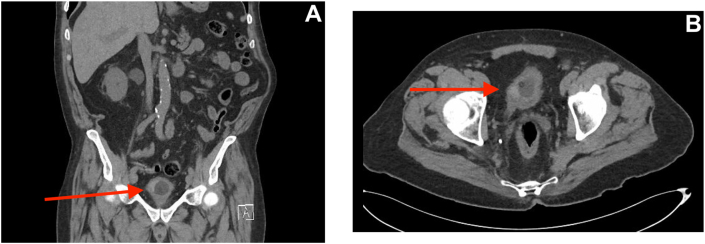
(A) Coronal non-contrast CT of the abdomen and pelvis demonstrating moderate dilation of the right collecting system and ureter with an obstructing lesion at the level of the right ureteral insertion and no mass or adenopathy at the site of prior left nephrectomy. (B) Axial non-contrast CT of the abdomen and pelvis demonstrating a mucosal-based obstructing lesion at the right ureteral orifice with associated upstream ureteral dilation.
